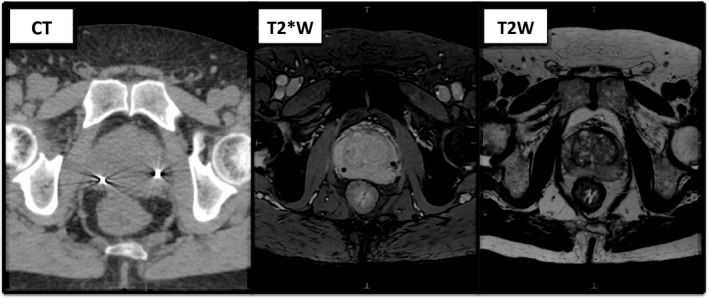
The three imaging sequences used for prostate contours showing the corresponding levels for the same patient. From left to right (a) CT imaging‐ fiducials seen as bright markers with surrounding artifact (b) T2*W MRI sequence‐ fiducials seen as dark void areas (c) T2W MRI sequence‐ fiducials not visible.
